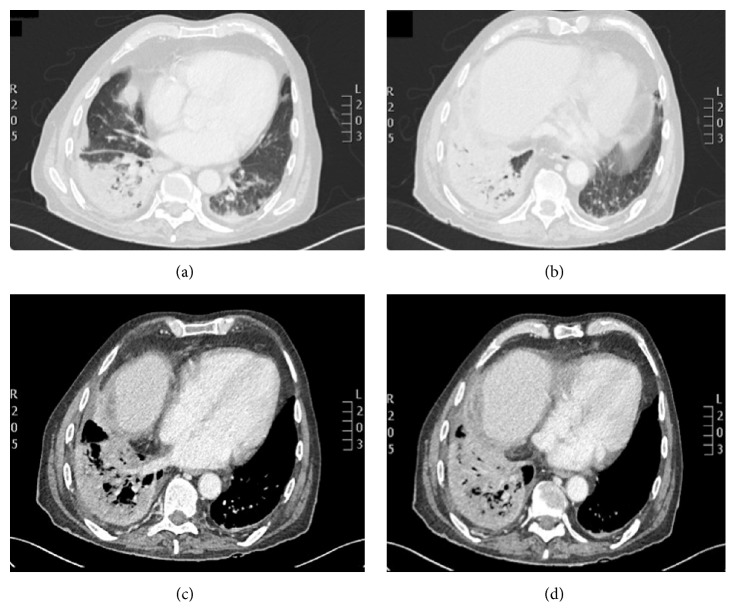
Computed tomography of the chest on admission. Right lower lobe alveolar consolidation with air bronchogram and in the left lower lobe and posterior segment of the left upper lobe similar lesions were identified in relation to a bilateral pneumonic process with associated loculated right pleural effusion and diffuse pleural thickening related to empyema.
